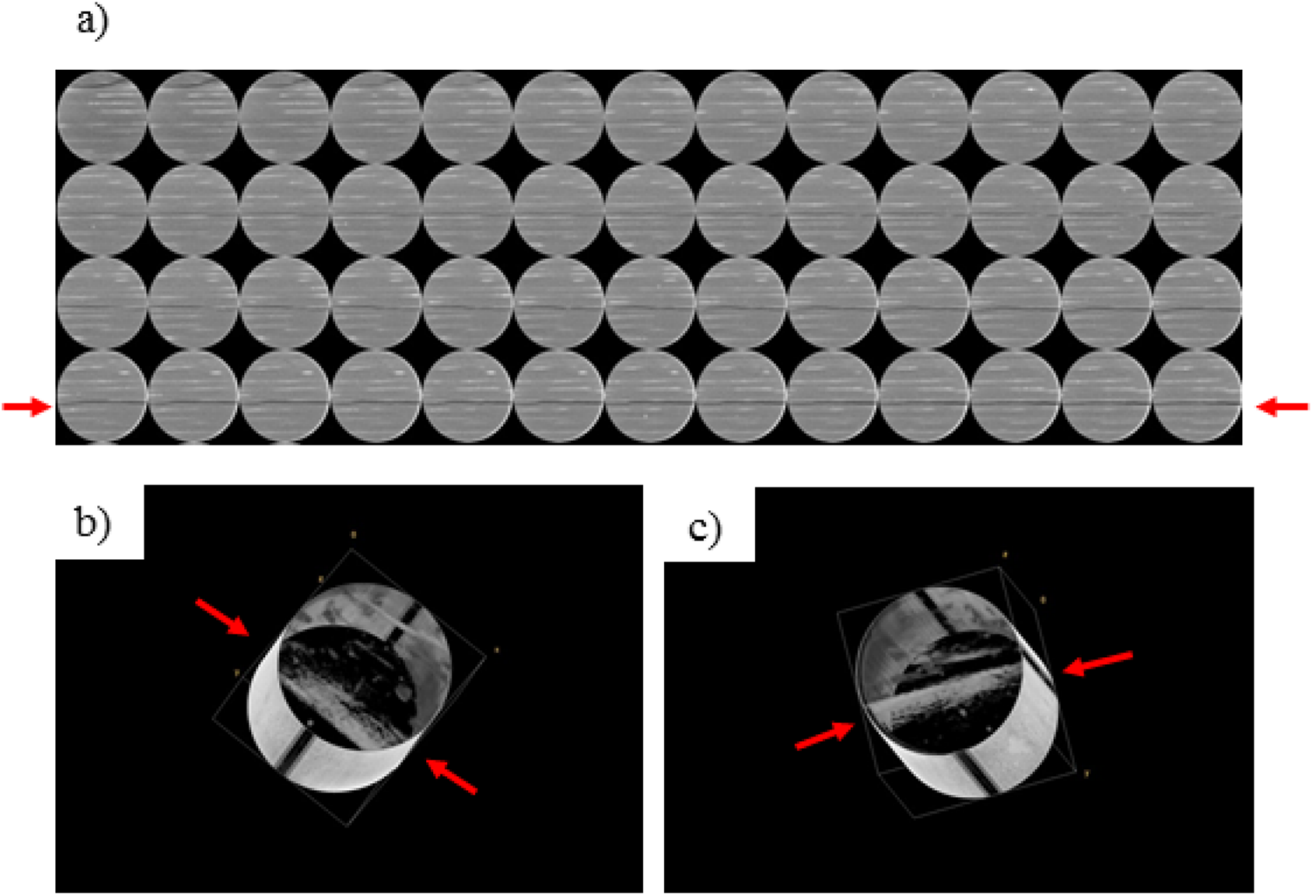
CT scanning images of 2D and processed 3D views of Duv 1–1: (a) 2D, top to bottom; (b) 3D, top to bottom; (c) 3D, bottom to top. A primary cemented lamination that is partly fractured is highlighted with the red arrows (a–c). There is a secondary fracture close to the edge (a,b) that is not continuous along the core axis. Drafted using ImageJ (version 1.53a, https://imagej.net/).
